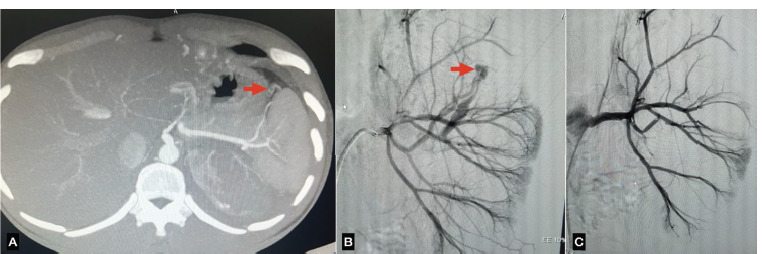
(A) CT Angiography showing an arteriovenous fistula in the right renal upper polar artery. (B) Diagnostic angiogram showing an arteriovenous fistula. (C) Post procedure angiographic image showing no evidence of AV fistula.
